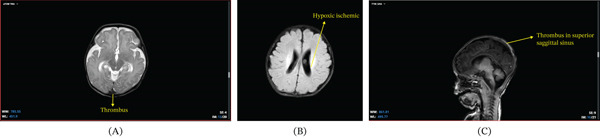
Representing the MRI report of Case 5: (A) thrombus, (B) hypoxic ischemic, and (C) thrombus in superior sagittal sinus.
